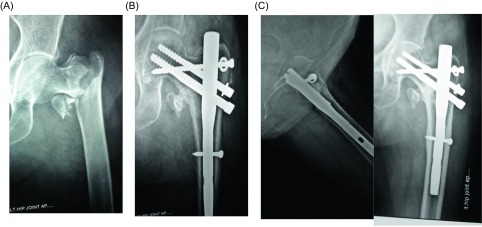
A 65-year-old male patient with a type 31-A3.3 fracture. (A) Initial radiograph. (B) Radiograph of the proximal femur at the 6th postoperative week with additional screw with washer. (C) Radiograph of the proximal femur at the 24th postoperative month showing complete consolidation of fracture.
